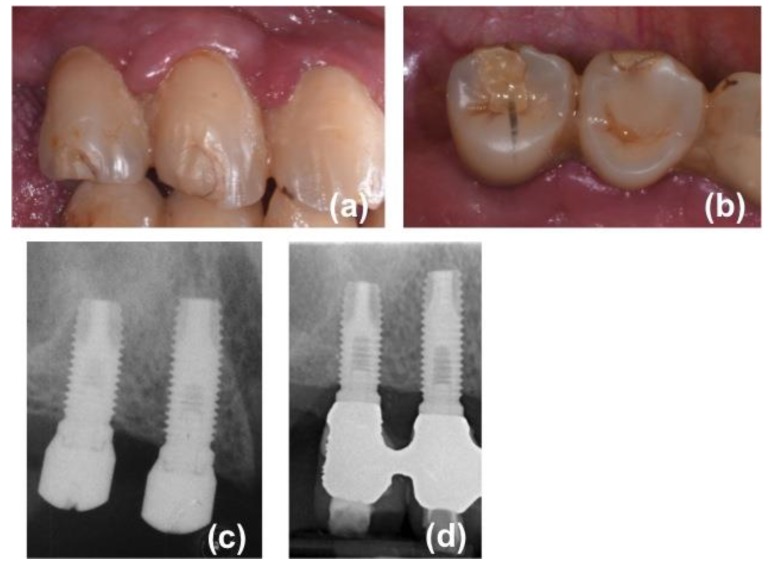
One of the patients included in the present research: (a) intraoral view of the bridge at the five-year follow-up appointment; (b) occlusal view of the bridge at the five-year follow-up appointment; (c) endoral radiograph three months after implant insertion; (d) endoral radiograph taken at the five-year follow-up appointment.
